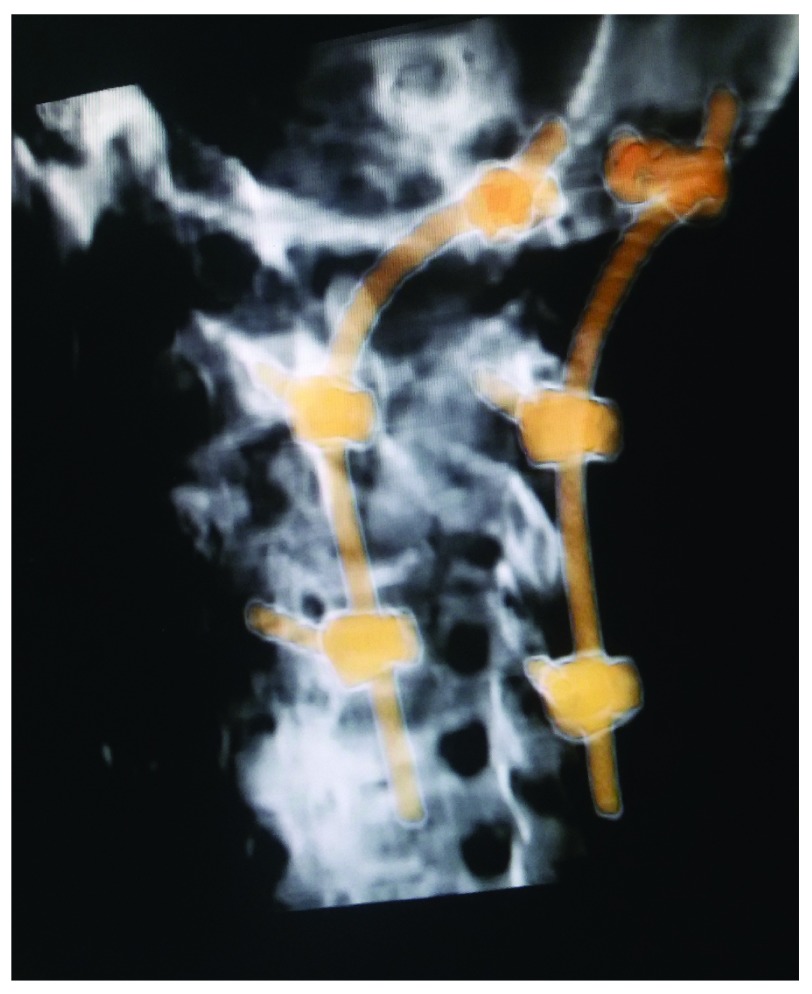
CT spine reconstruction showing projection and final alignment of the construct.
